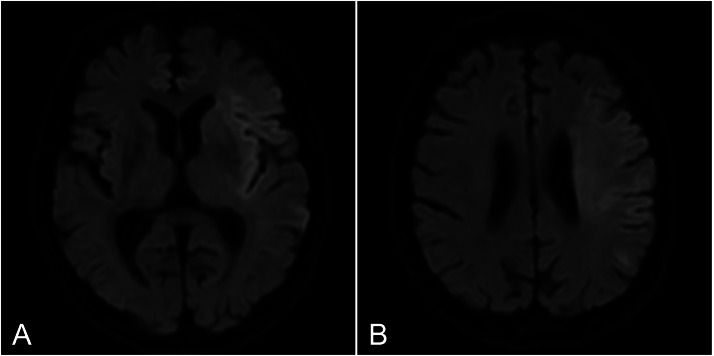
Magnetic resonance imaging findings at admission. (A, B) Diffusion-weighted images showing acute cerebral infarcts in the left middle cerebral artery territory.
